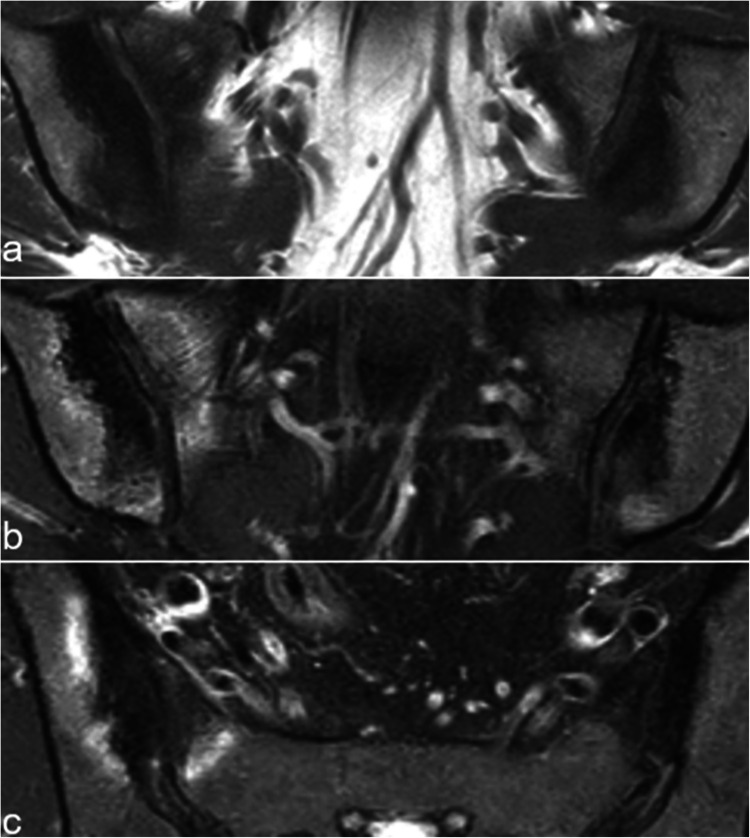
A 37-year-old woman with OCI. Coronal oblique a T1- and b FS T2-weighted images. c Axial oblique FS T2-weighted image show bilateral extensive hypointense subchondral sclerosis on the iliac side, which is well delineated, and surrounded by a mild rim of BME on the right side. Similar changes are observed on the sacral side. These findings predominate at the anterior aspect of the middle third of the SIJ
